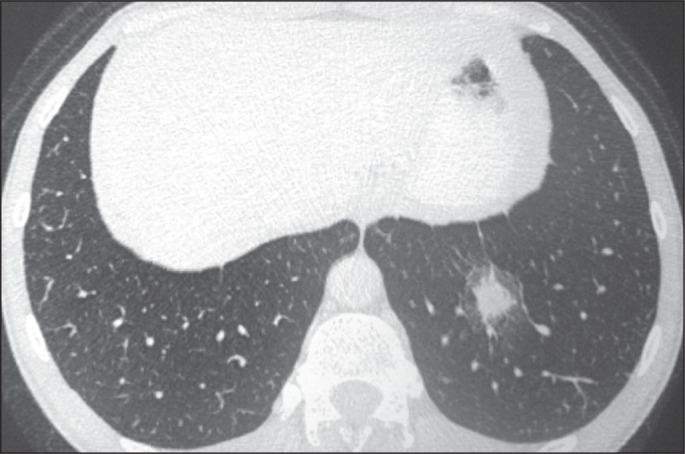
CT of a 47-year-old man with positive serology, showing a nodule
